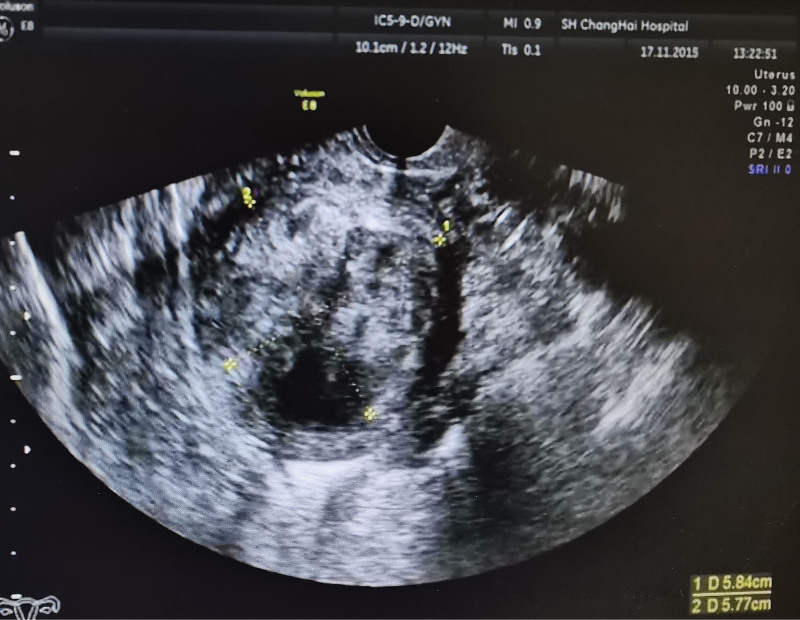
Transvaginal ultrasound at the patient's first visit in 2015. The diameter of uterine leiomyoma was about 5.8 cm.
